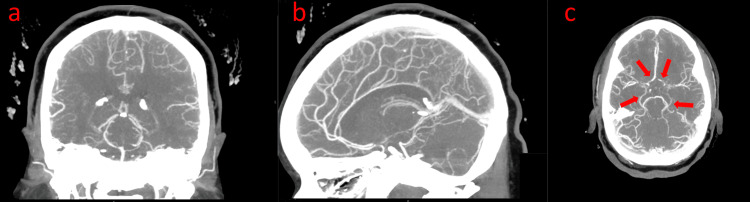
CT angiogram head and neck: moderate to severe narrowing at the origins of the bilateral MCA M1 segment and ACA A2 segment, which were patent distally. (a) Coronal view, (b) sagittal view, and (c) transverse view (red arrows identifying affected areas) CT: computed tomography; MCA: middle cerebral artery; ACA: anterior cerebral artery
